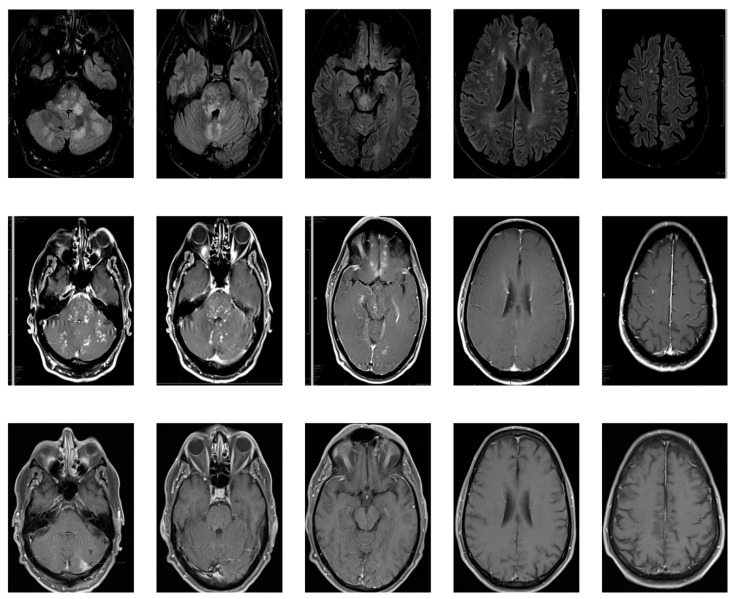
MRI showing Multifocal enhancement of the brain and spinal cord. Striking abnormal patchy and rounded increased T2 signal, predominantly in the brainstem, involving the medulla and the pons, middle, right, and inferior cerebellar peduncles. There is clear involvement of the midbrain and cerebral peduncles. There is involvement of the medial orbitofrontal white matter greater than gray matter and relative sparing of temporal lobes but notably there are supratentorial <3 mm lesions relatively symmetric and some of which contrast-enhance. There is no diffusion restriction. There is minimal mass effect or edema. Row 1: T2 FLAIR; Row 2: T1 post-contrast at presentation; Row 3: T1 post-contrast 20 days after presentation and post-steroids.
